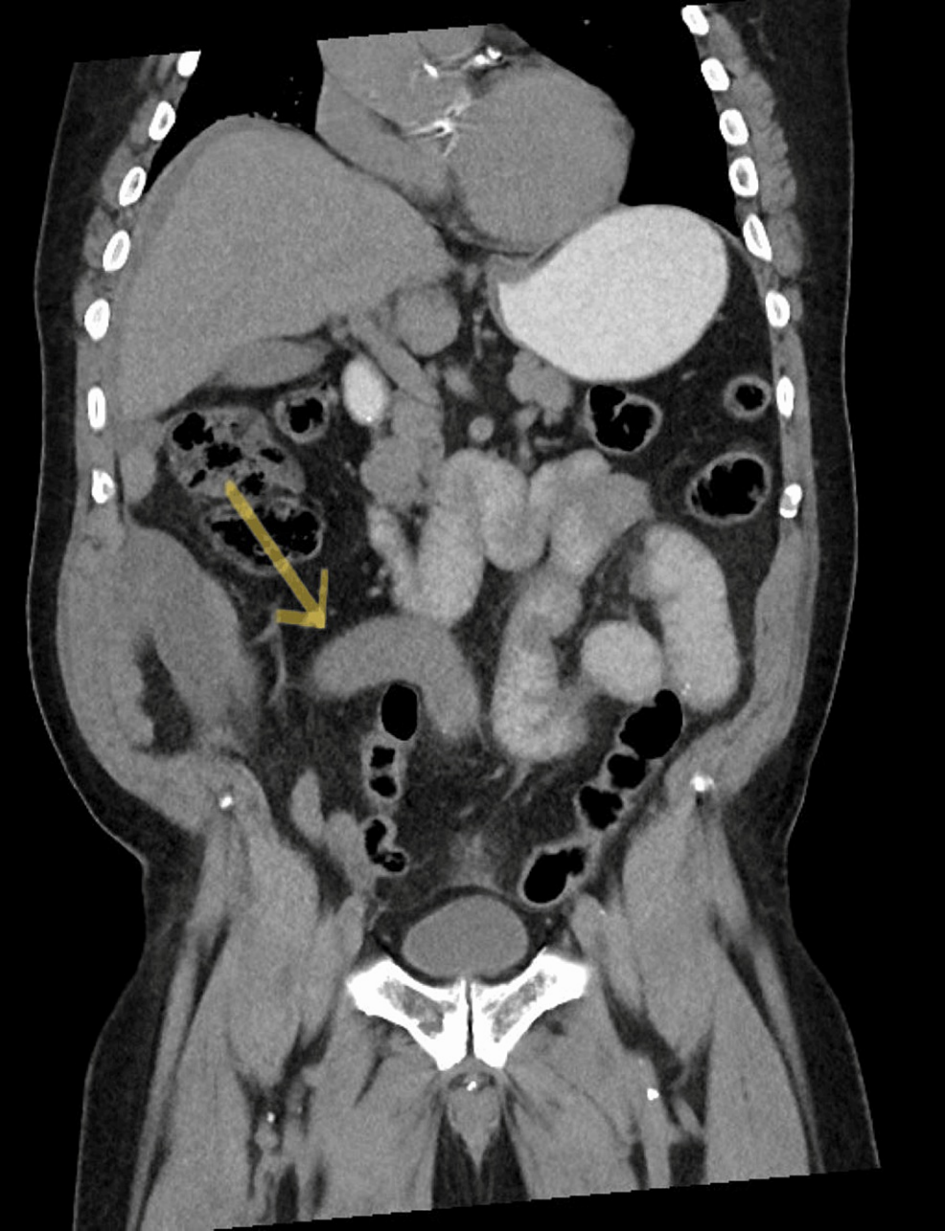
Coronal CT abdomen/pelvis with oral contrast showed mild small bowel dilation with a transition point (see arrow) lateral and anterior to the right paracolic gutter. No distal oral contrast is noted.
